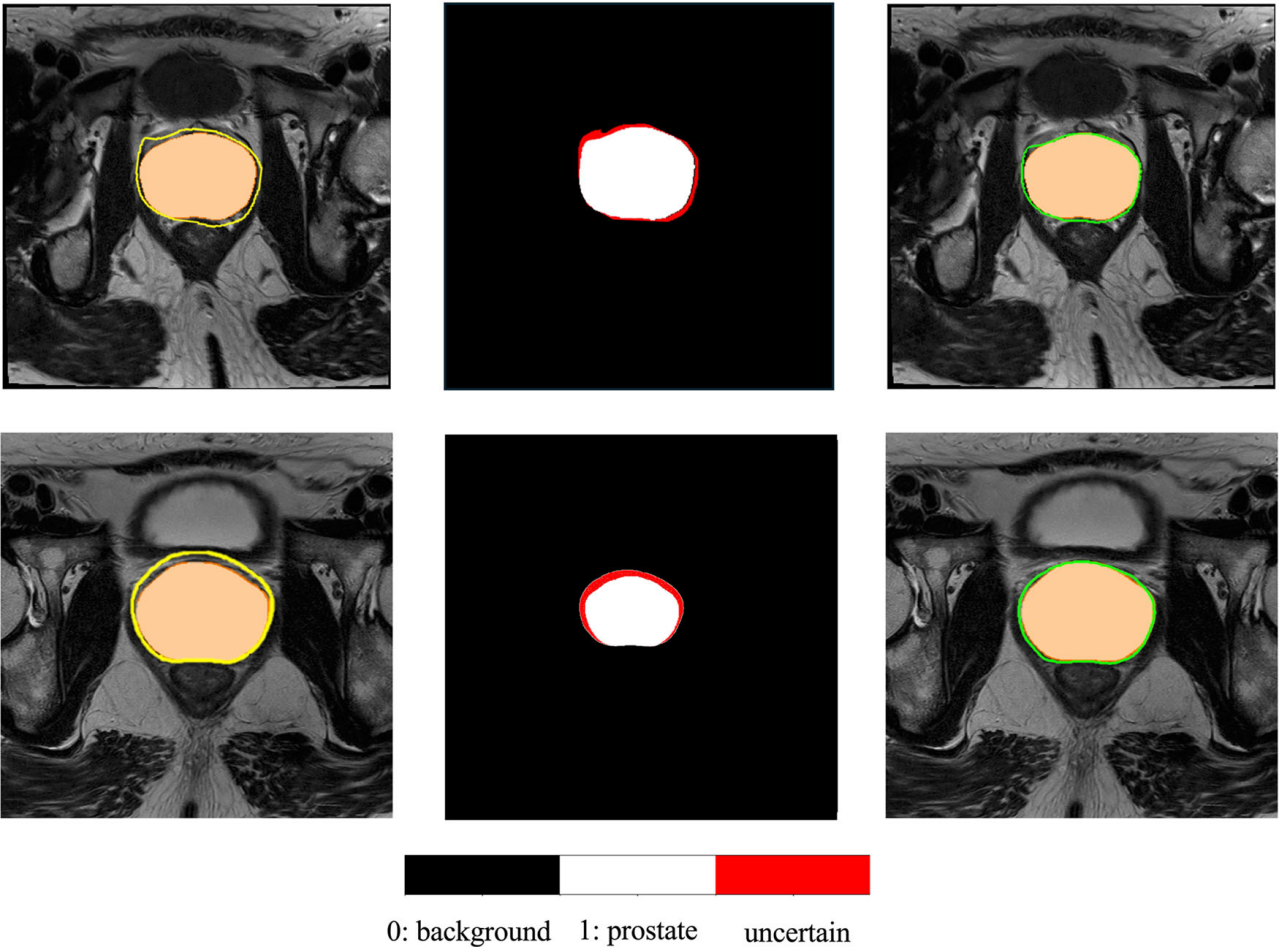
In each row, resulting segmentations for one slice from two different T2w patient MRI exams. The left column is outlined prostate contour resulting from the deep-learning algorithm (yellow), and in the middle is the result of applying the conformal prediction to the output of the deep-learning algorithm. In the right column, outlined prostate contour resulting from withholding pixel predictions flagged as unreliable by the conformal predictor. Conformal prediction was applied with a confidence level of 85%
